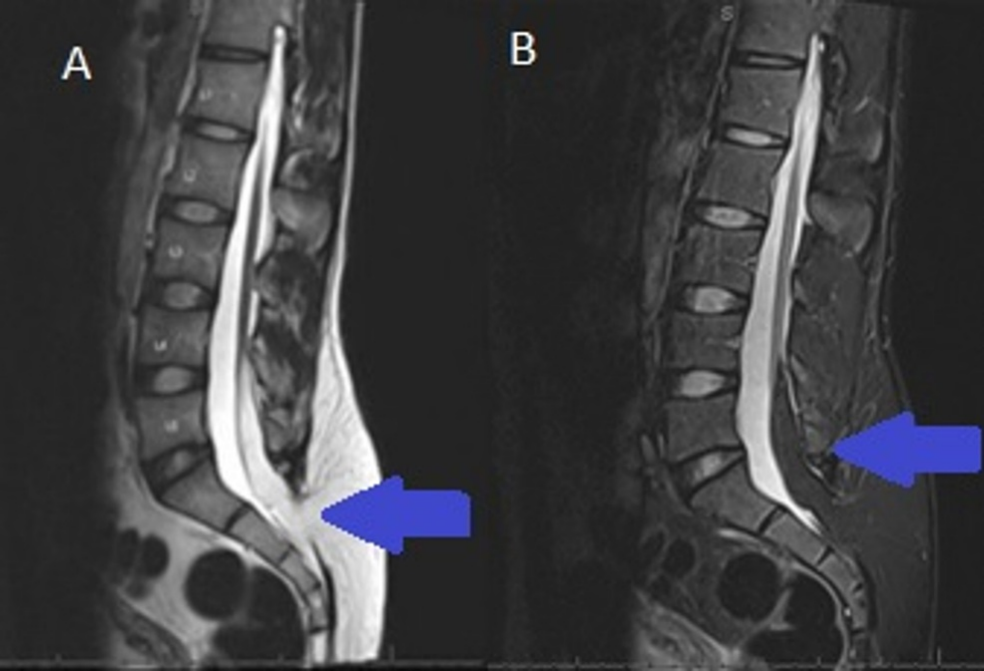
Lumbar spine sagittal view showing findings of tethered spinal cord with associated posterior sacral defect and lipomyelomeningocele MRI lumbar spine (A) T1 sequence and (B) T2 sequence show posterior sacral defect and lipomyelomeningocele (blue arrow).
